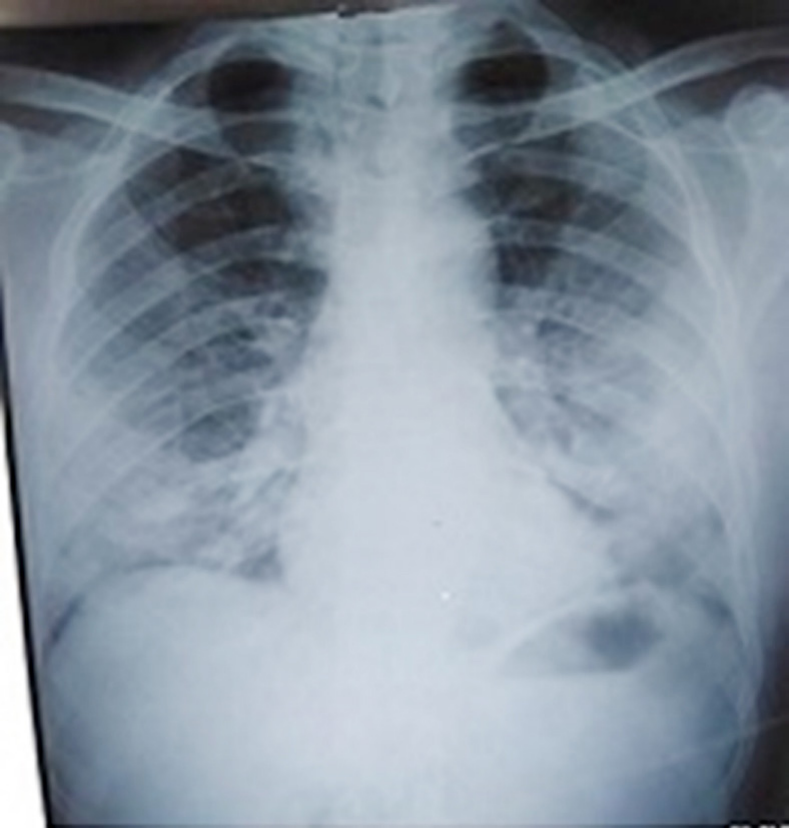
Chest X-ray of a seven years old female child showing Extensive Bilateral peripheral consolidation with air bronchograms predominantly right side, partially obscured right diaphragmatic silhouette and obscured ipsilateral CP angle. Radiographic findings are indeterminate for COVID-19. She had strong contact history, presented in ER with acute complains of SOB, cough, fever and flu.
